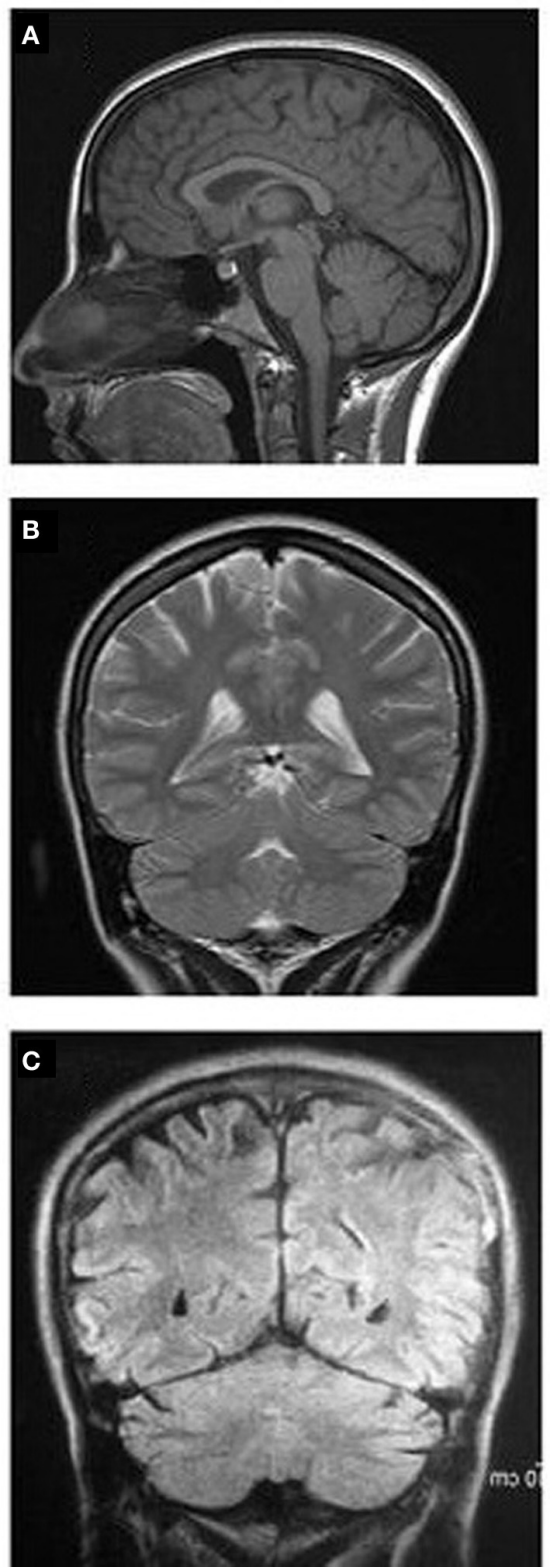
MRI images showing sagittal (A) and coronal (B) sections of case 1 with facultative QL; (C) coronal MRI of case 2 with a paralyzed leg. Notice the entirely normal brain structures in both cases.
